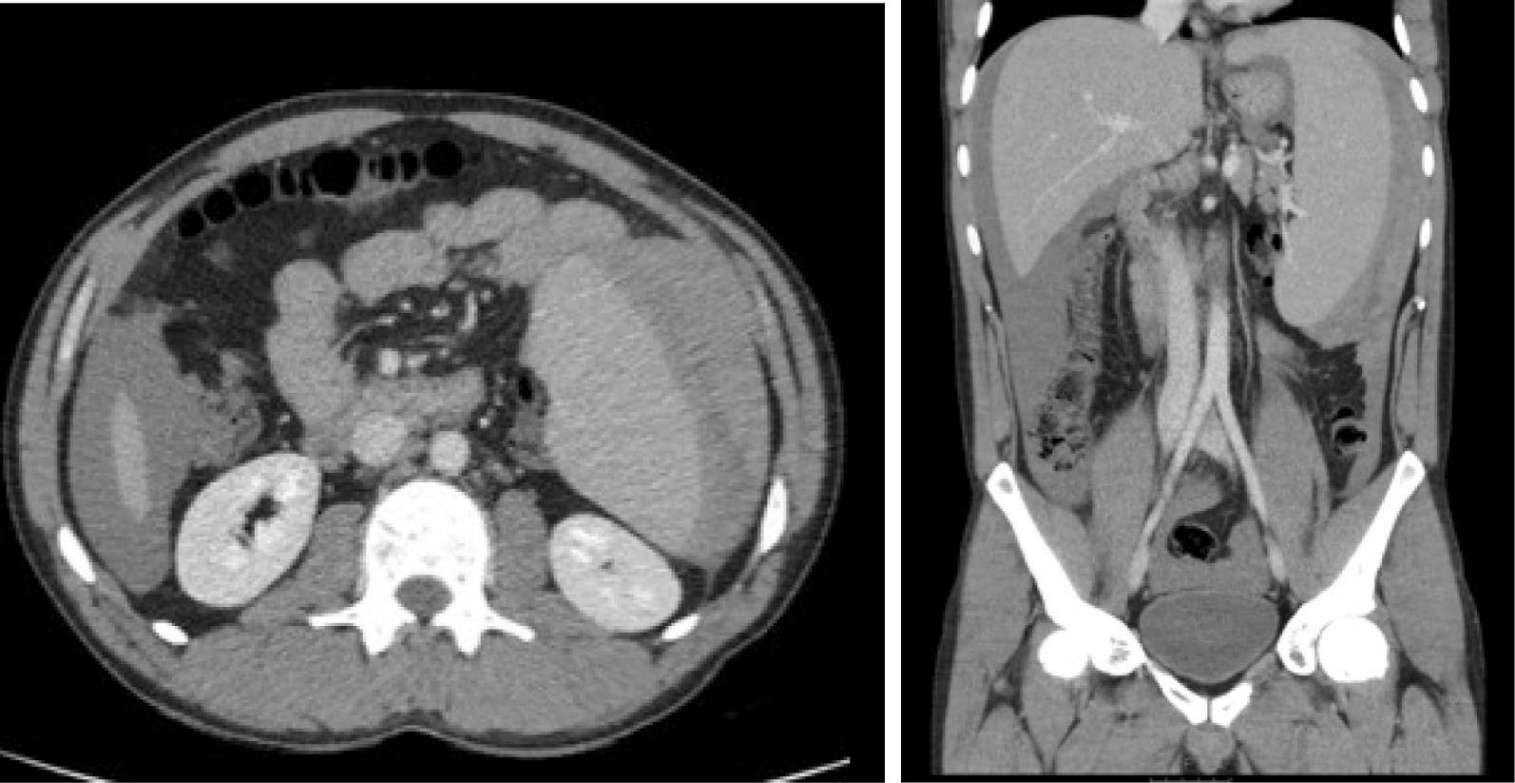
CT abdomen (axial and coronal views) with contrast showing splenic rupture, splenomegaly, and intra-abdominal haemorrhage
